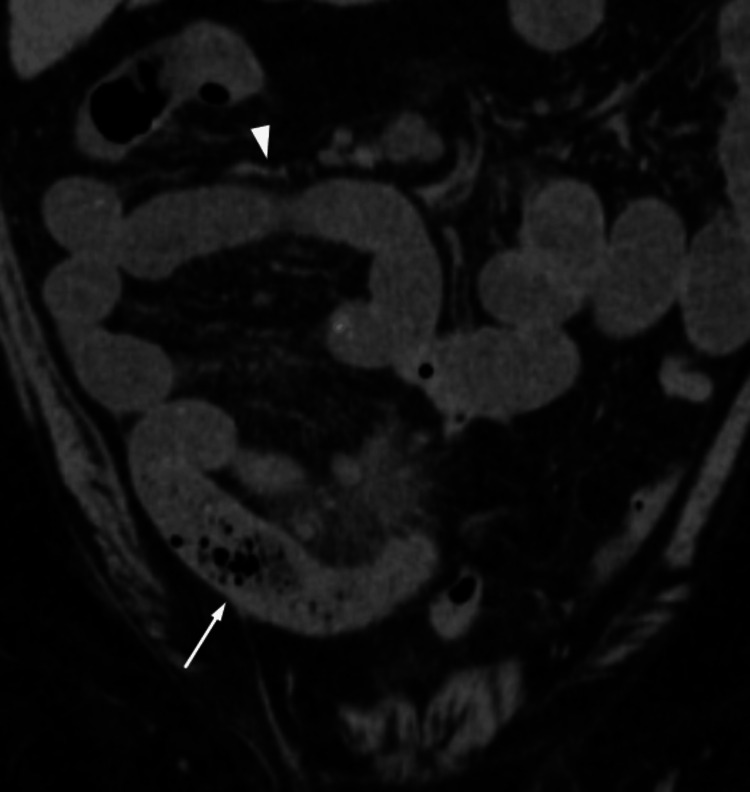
Abdominal CT scan image in coronal view showing dilated small bowel loops (white arrowhead) with the small bowel feces sign (white arrow). CT: computed tomography
